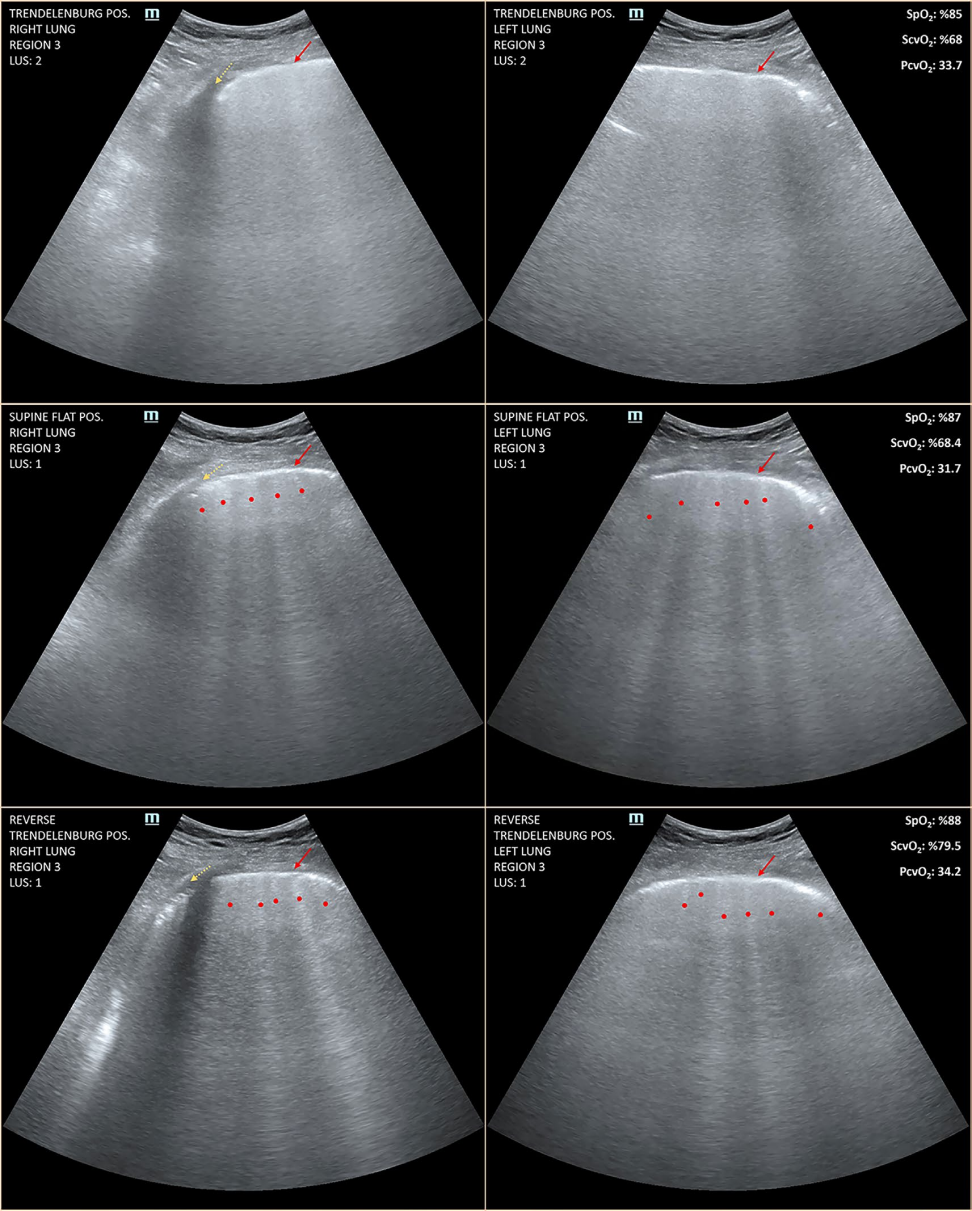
Ultrasonographic findings corresponding to loss of aeration with the position. At region 3, LUS score was 2 (white lung) for both lungs in the Trendelenburg position; 1 (well‐separated B‐lines) for both lungs in the supine flat position; and 1 (well‐separated B‐lines) for both lungs in the reverse Trendelenburg position. The red arrow indicates pleural line; the yellow arrow indicates pleural effusion; the red dots indicate for B‐lines. LUS score, lung ultrasound score; PcvO_2_, central venous O_2_ pressure; ScvO_2_, central venous O_2_ saturation; SpO_2_, peripheral O_2_ saturation. Consent for publication of the ultrasound images was obtained from the patient's legal representative.
